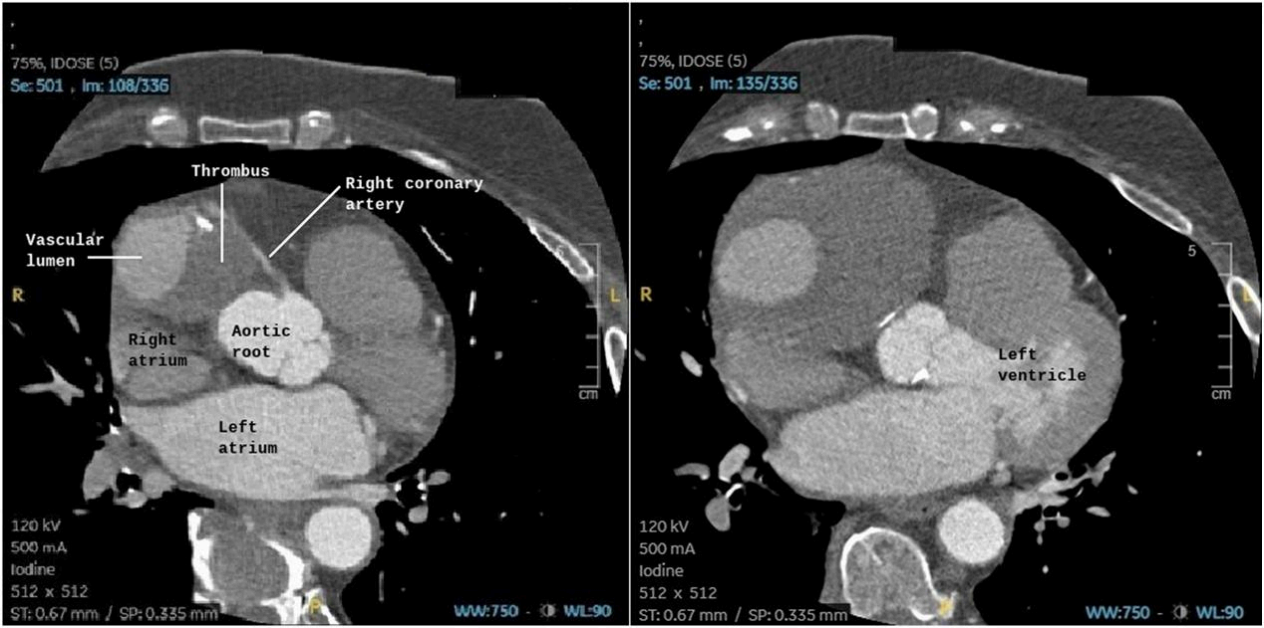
Axial coronary CTA showing a mass with focal contrast enhancement during the arterial phase and a peripheral non-enhancing region suggestive of thrombus along the course of the right coronary artery. The mass partially compresses the right atrium and is consistent with a giant aneurysm.
